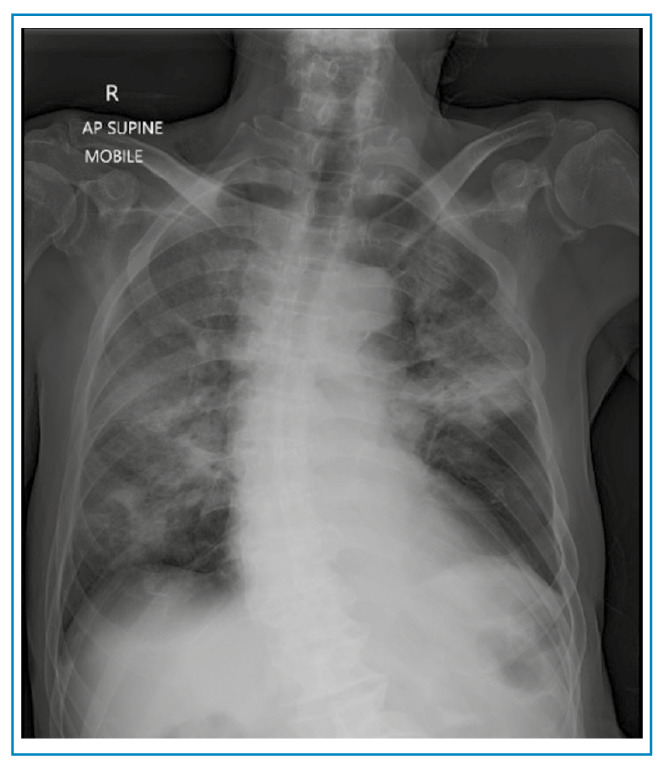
Chest radiograph on admission showing bilateral diffuse ground-glass opacities.
